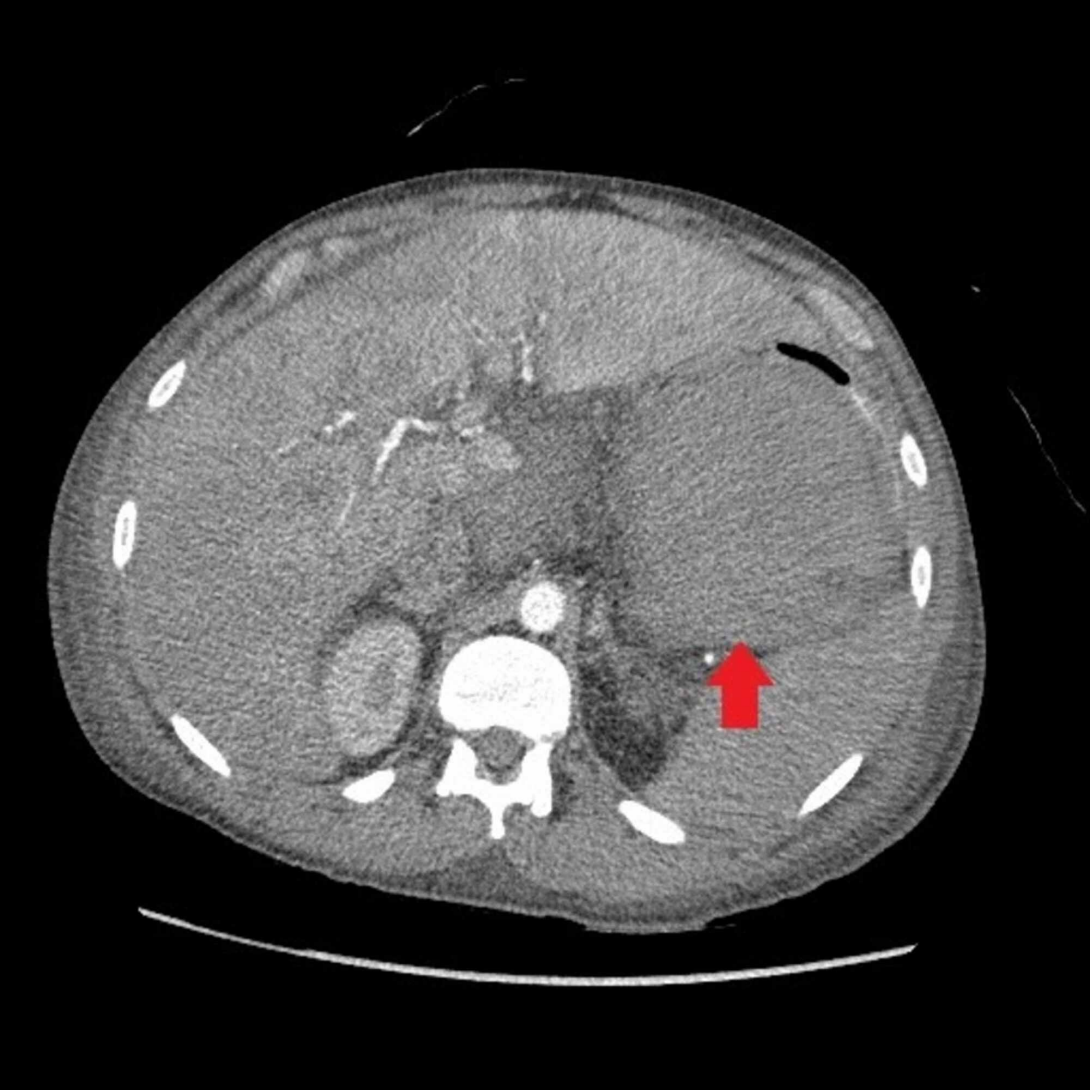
Axial abdominal CTA showing retro gastric intraperitoneal hematoma compressing the stoma CTA: computed tomography angiogram.
